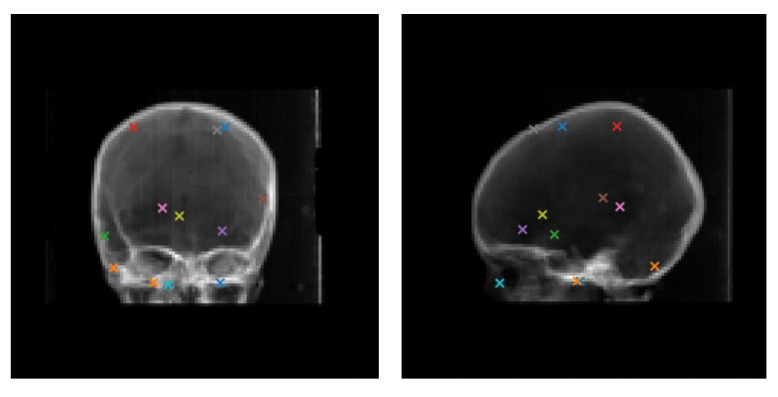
A example of 12 landmarks in head CT image.
